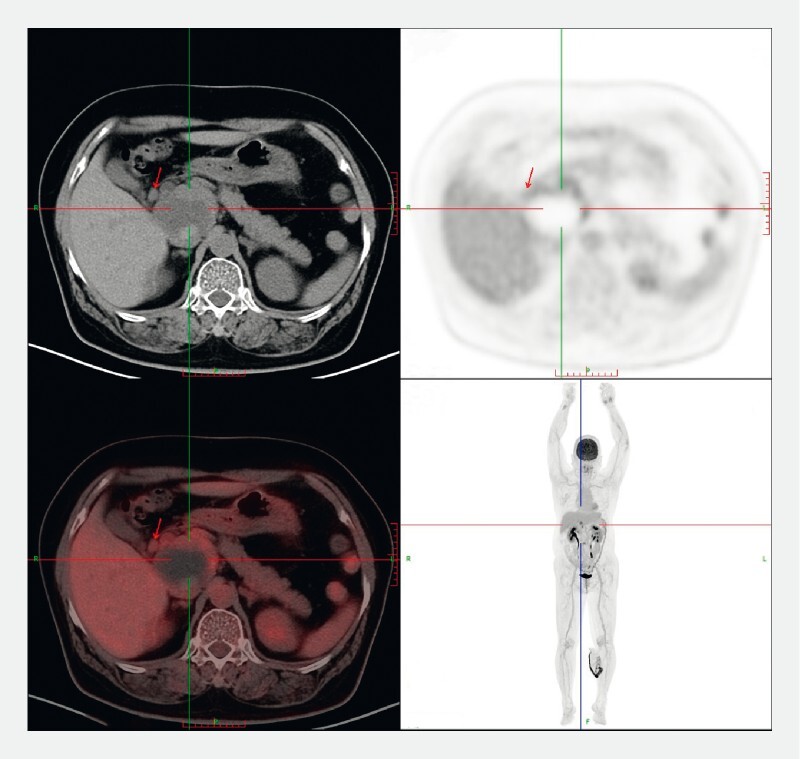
Positron emission tomography/computed tomography of the mass.
